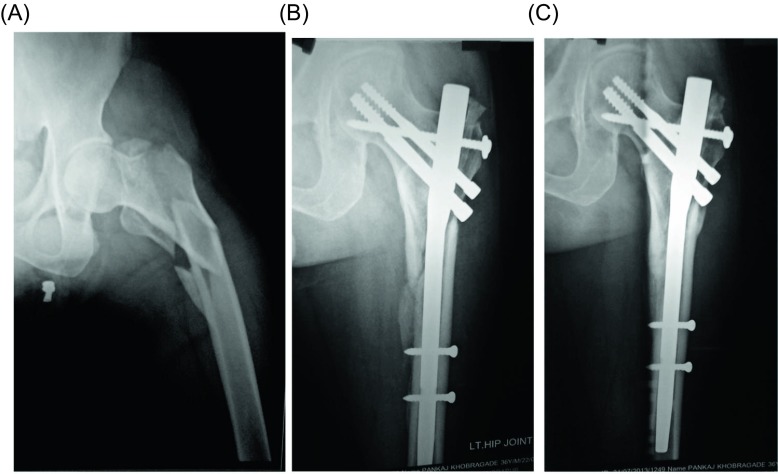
A 72-year-old female patient with a type 31-A3.3 fracture. (A) Preoperative Initial radiograph. (B) Radiograph of the proximal femur at the 6th postoperative week with additional screw with washer. (C) Radiograph of the proximal femur at the 12th postoperative month showing good consolidation of fracture.
